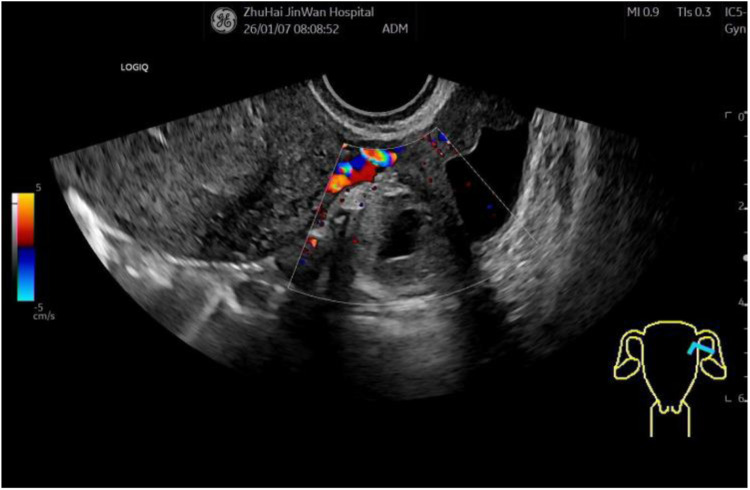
Transvaginal ultrasound on day 5 of treatment (January 7, 2026). The ectopic mass transiently enlarged to 24 × 20 mm with an irregular gestational sac, suggesting necrosis and hemorrhage.
